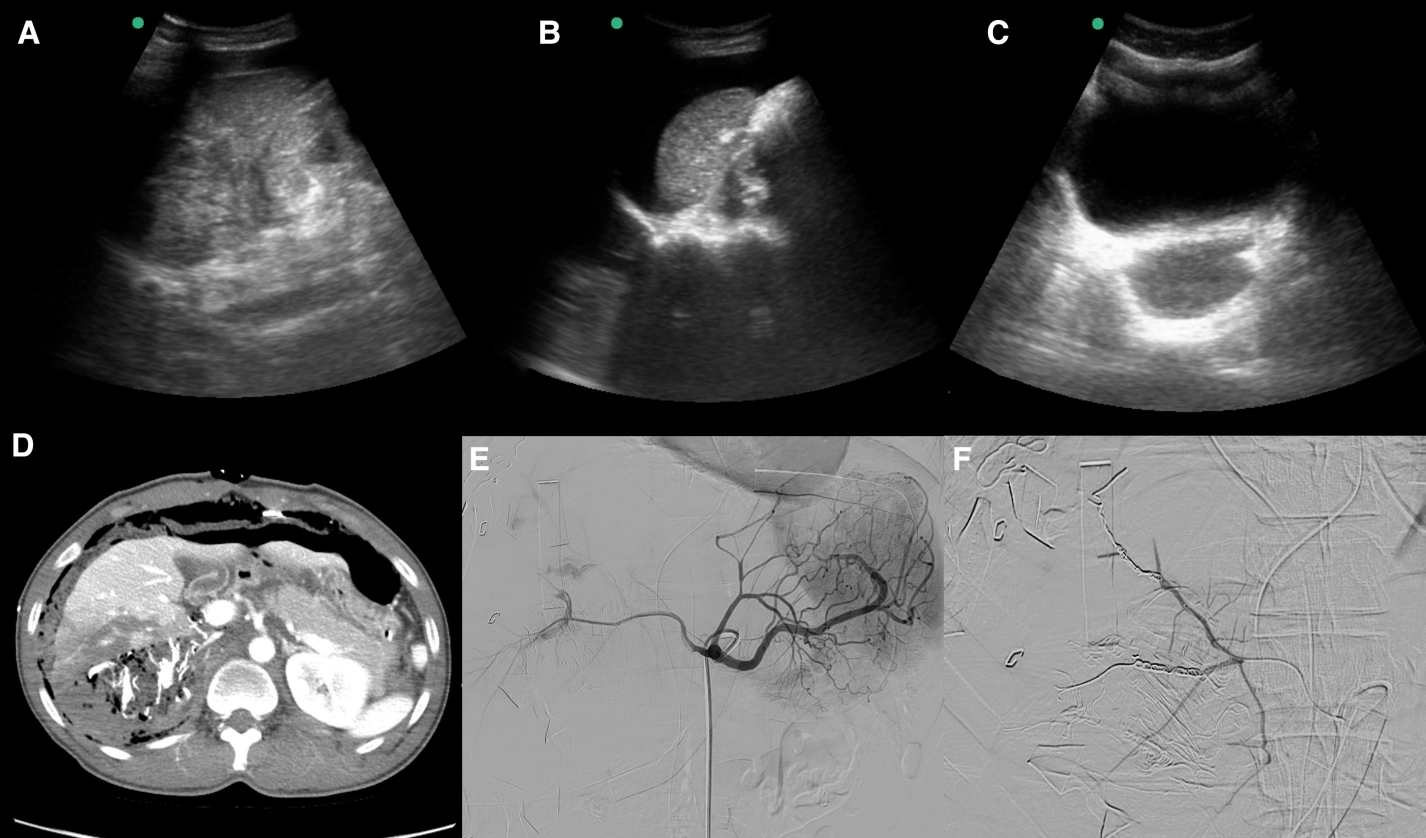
Focused assessment with sonography in trauma (FAST), postoperative computed tomography (CT), and angioembolization in a patient with a blunt retrohepatic vena cava injury. (A) FAST (Morrison's pouch, no free fluid). (B) FAST (perisplenic free fluid). (C) FAST (pelvic cavity, no free fluid). (D) Postoperative abdominal contrast-enhanced CT. (E,F) Postoperative angioembolization.
